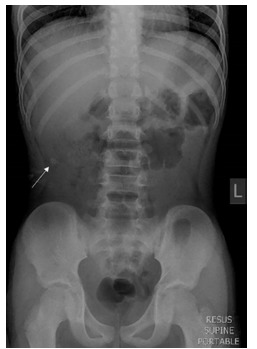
Abdominal radiography showing a circular radiopaque structure (arrow) over the right hypochondrium.
